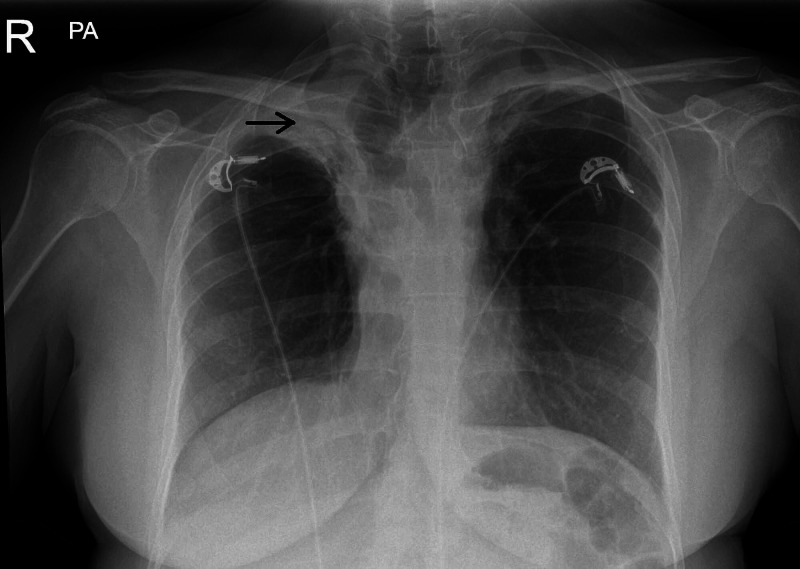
Chest X-ray film showing extensive volume loss in both upper lobes, more marked on the right side and tracheal deviation to the right, secondary to previous mediastinal radiotherapy (arrow)
